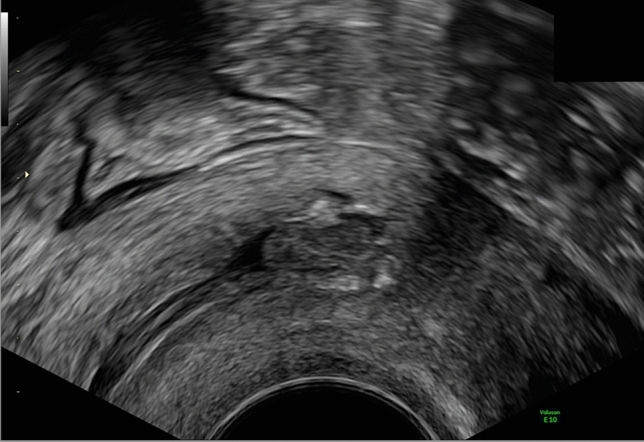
HYPERLINK "sps:id::fig1||locator::gr1||MediaObject::0" :Transvaginal sonogram shows a polyp located at the cervicouterine junction
